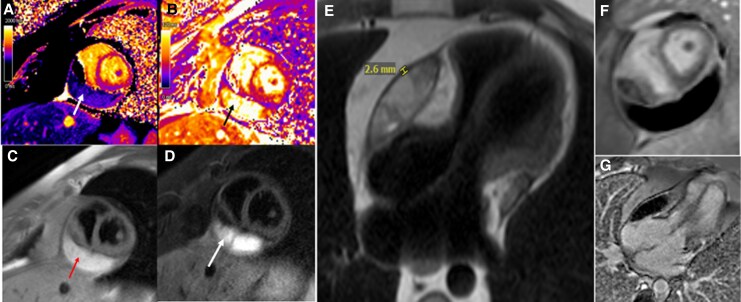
Cardiac MRI demonstrated a pericardial fluid collection with T1 mapping showing shorter relaxation time than myocardium (panel *A*) and corresponding hyperintensity on T1-weighted fat-suppressed imaging (panel *C*). T2 mapping revealed a longer relaxation time than myocardium (Panel *B*) with hyperintensity on T2-weighted STIR imaging (Panel *D*), without evidence of pericardial oedema. A loculated pericardial effusion with maximum pericardial thickness of 2.6 mm was visualized (Panel *E*). Phase-sensitive inversion recovery late gadolinium enhancement sequences showed no myocardial or pericardial enhancement (Panels F, G).
